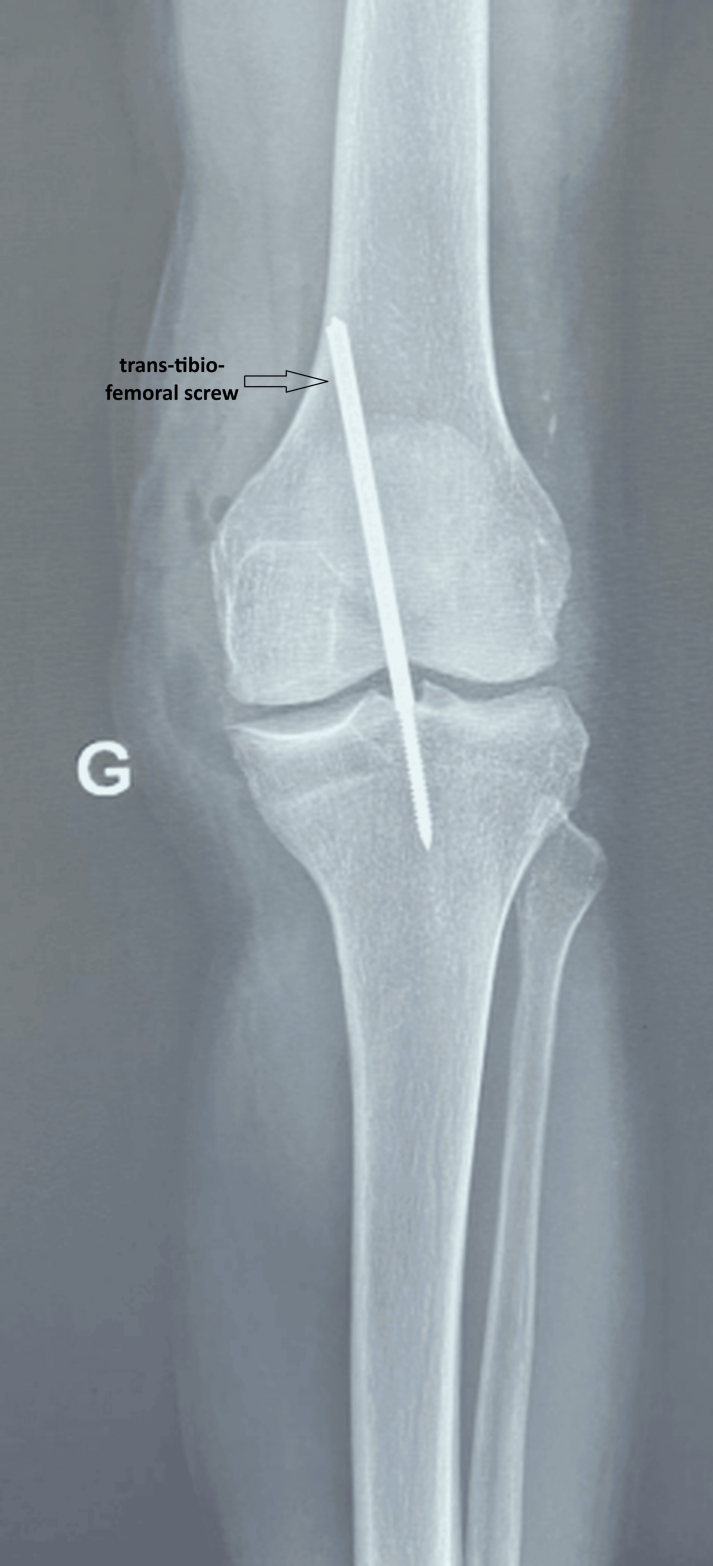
Standard radiograph of the left knee after reduction and stabilization with a trans-tibio-femoral screw
